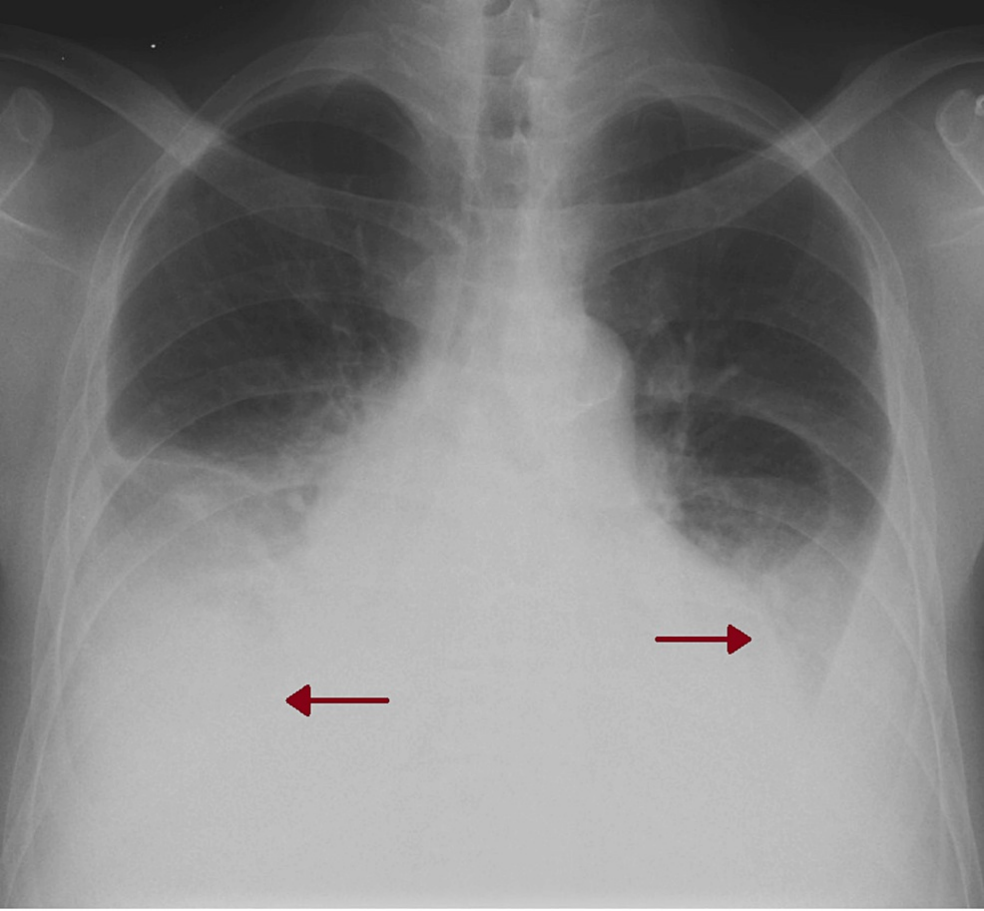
Second chest x-ray at a hemodialysis clinic (after two months) Red arrows: bilateral pleural effusion
